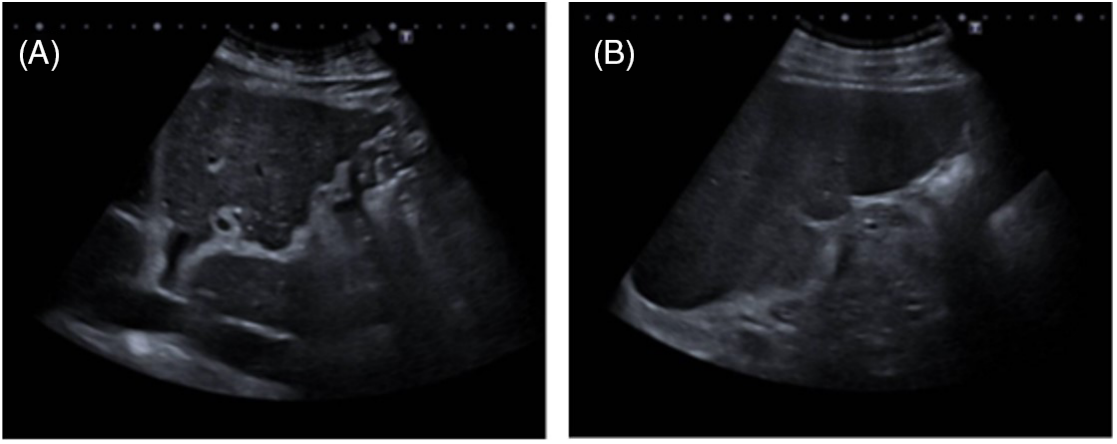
Liver ultrasound. (A) Cirrhotic liver with enlargement of the left lobe and periportal fibrosis. (B) Splenomegaly of 17 cm in longitudinal diameter
